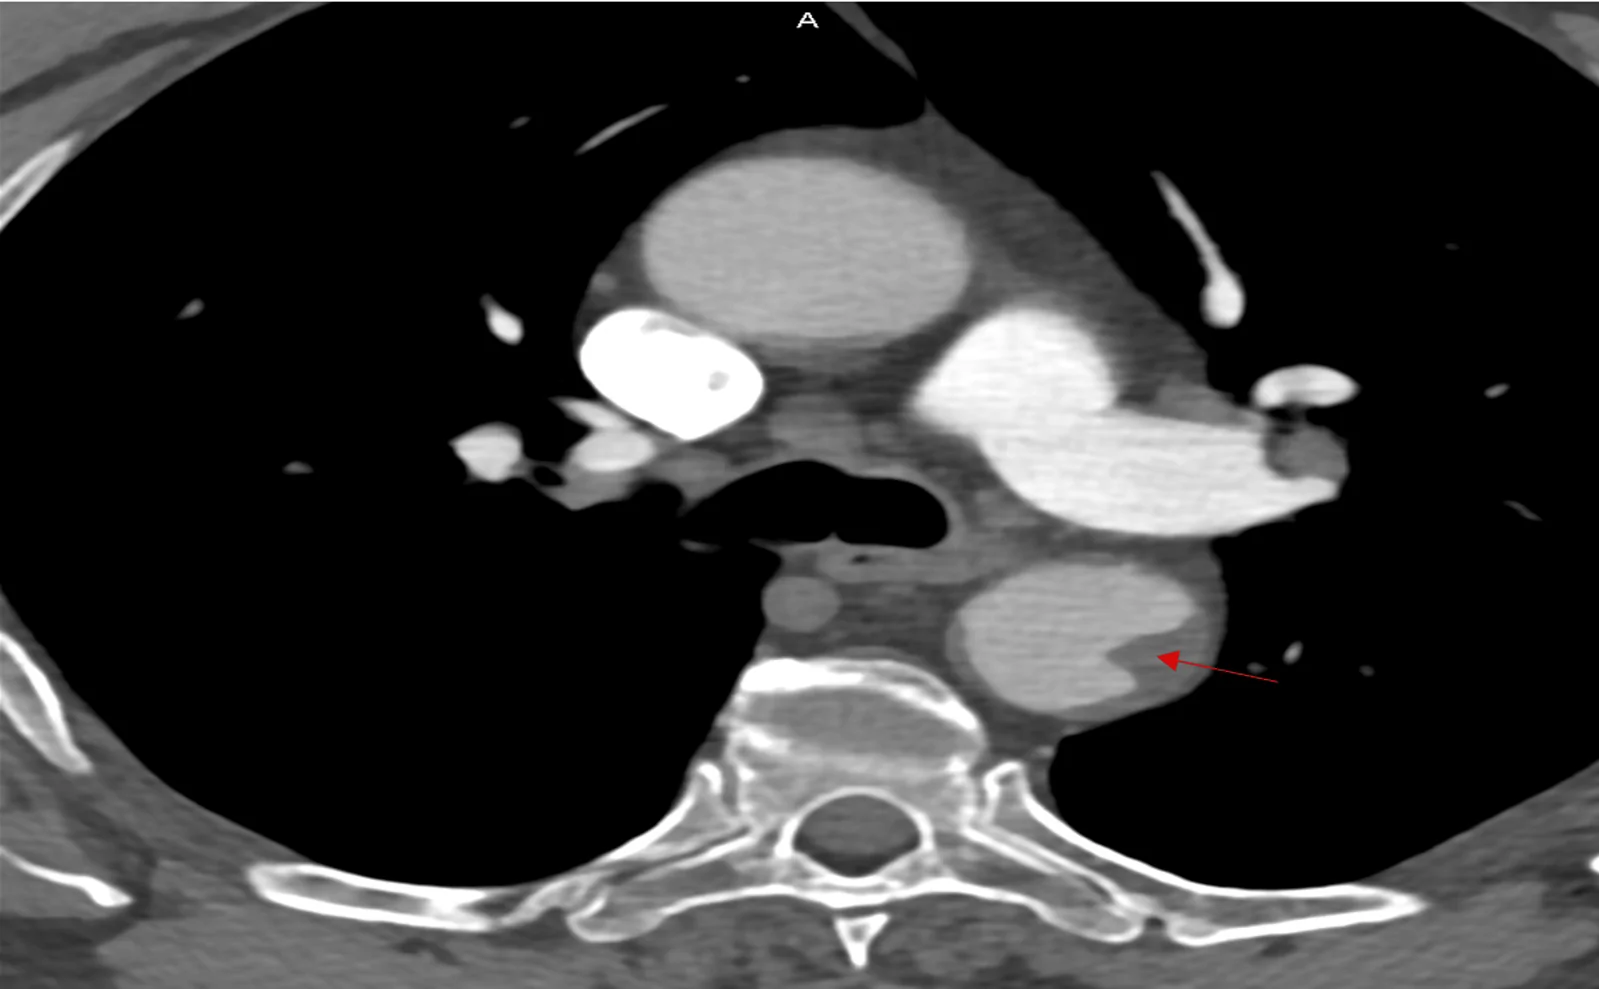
Computed tomography image showing an atheromatous plaque in the descending thoracic aorta (red arrow)
